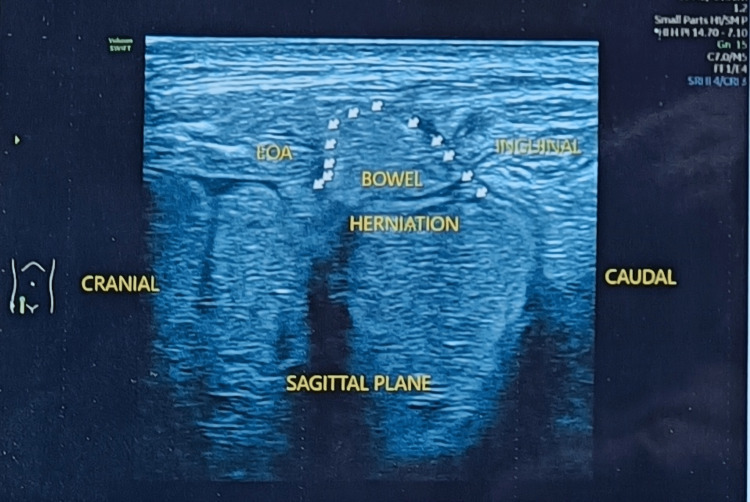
Ultrasonography of Spigelian hernia performed while in a standing position shows herniating bowel loops (EOA) indicated by multiple small white arrows EOA: External oblique aponeurosis
